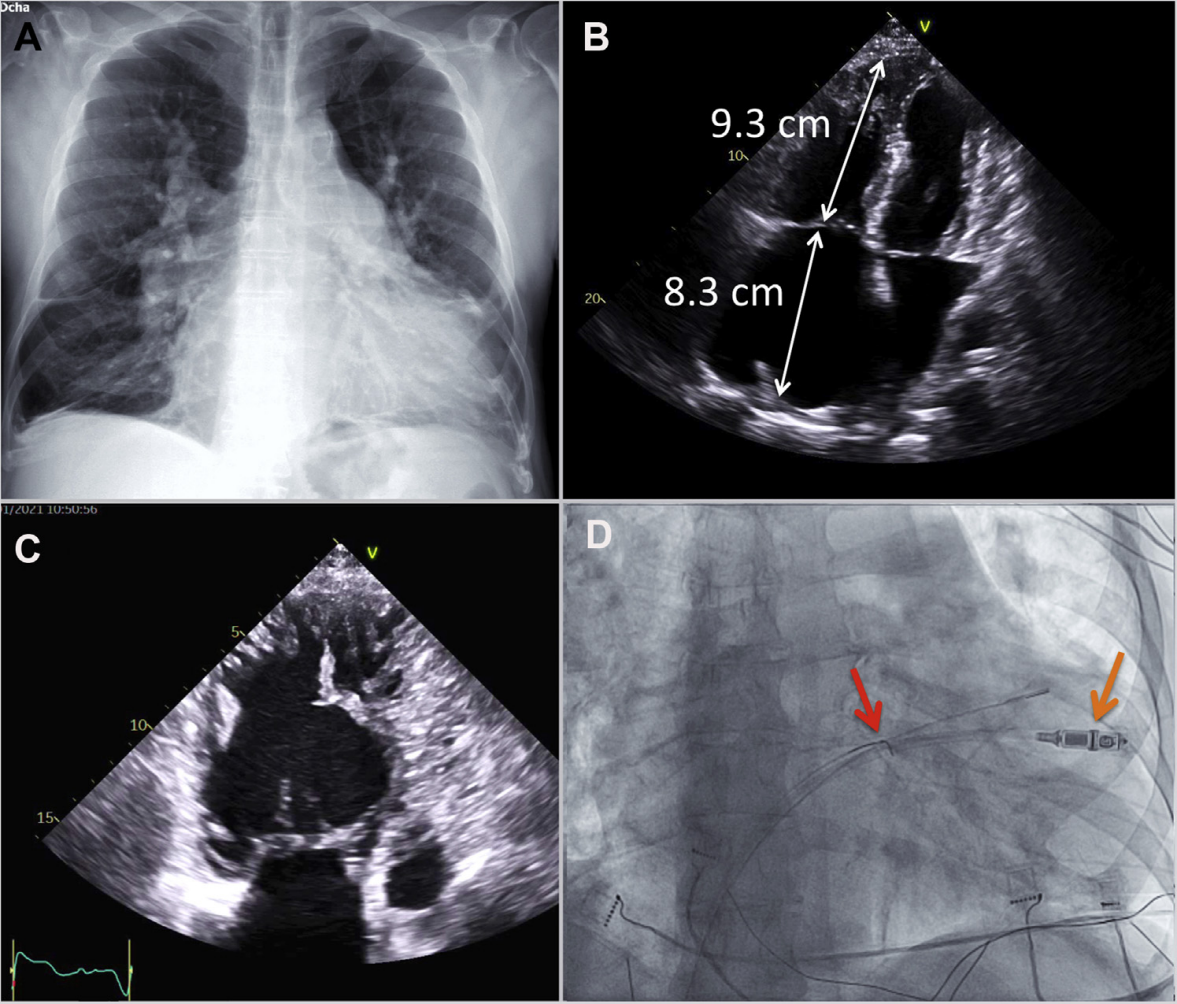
A: Posteroanterior thoracic radiograph with typical pulmonary arterial hypertension findings. B: Echocardiography 4-chamber view with severe right dilated chambers. Right ventricle: 9.3 cm. Right atrium: 8.3 cm. Right atrium area: 53.3 cm^2^. C: Modified apical 4-chamber view with right ventricle hypertrophic trabeculae. D: Fluoroscopic right anterior oblique view during leadless pacemaker (LPM) implantation with an Amplatz Gooseneck snare (Medtronic, Minneapolis, MN). *Red arrow:* Amplatz Gooseneck snare. *Orange arrow:* LPM deployment.
